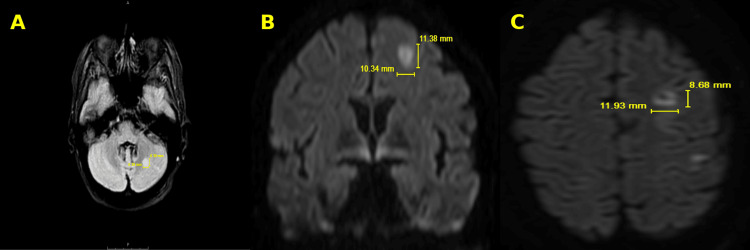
MRI brain imaging: lesion mapping with dimensions. Small focal areas of restricted diffusion in the left frontal lobe cortex near the vertex (A & B). Focal ill-defined T2 fluid-attenuated inversion recovery (FLAIR) hyperintense signal abnormalities in the left cerebellum (C).
